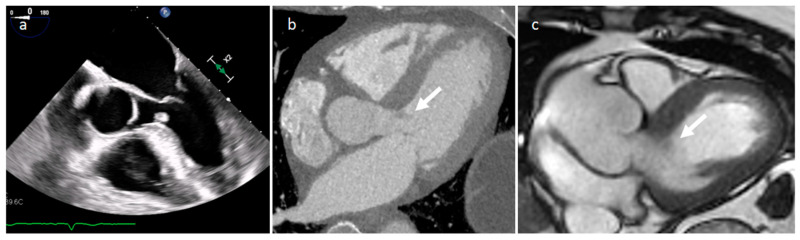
A left ventricular outflow tract fibroelastoma of about one centimeter during transesophageal echocardiography (a), CT (b) and cine MR imaging (c). These images show that a fibroelastoma can be more easily evaluable during ultrasonography.
